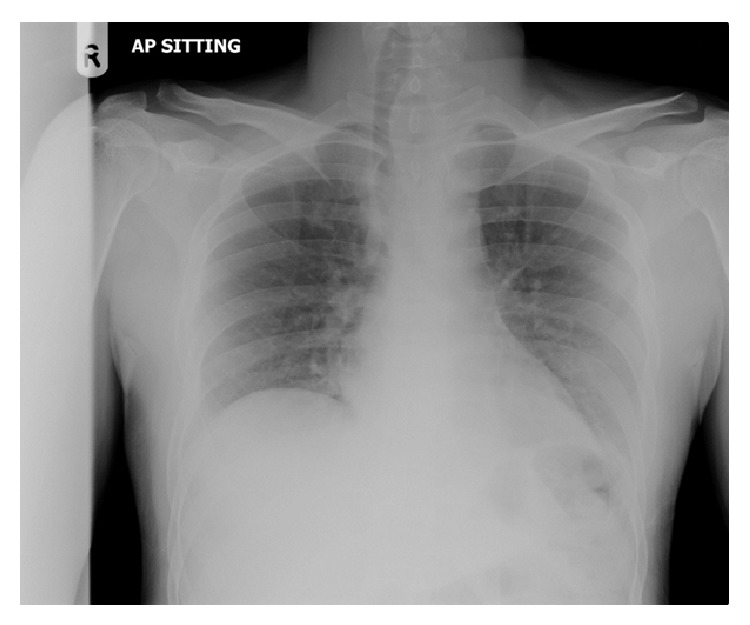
Chest radiograph showing tracheal deviation but otherwise unremarkable.
